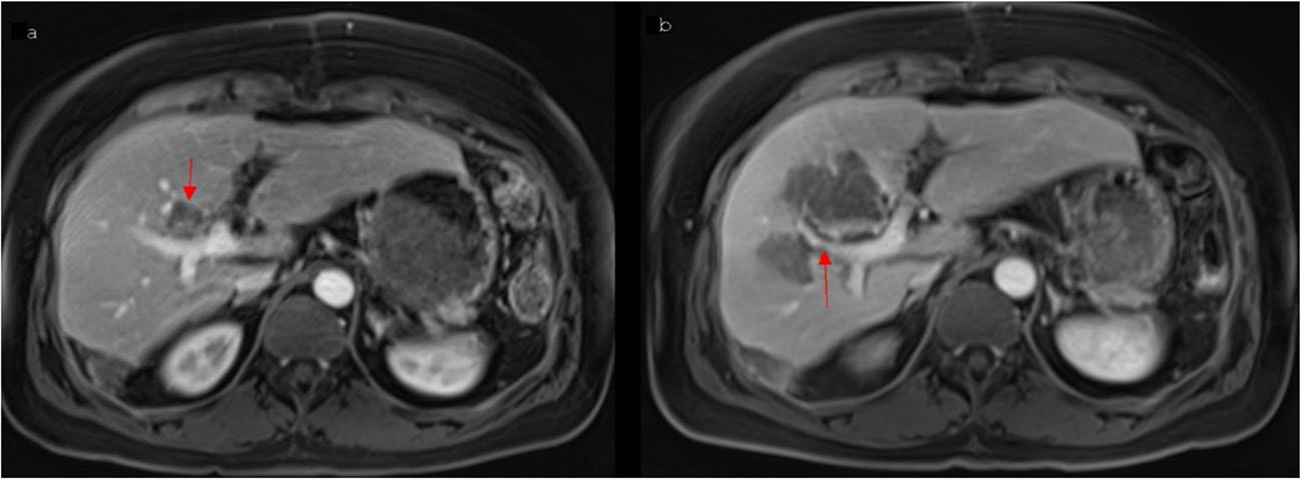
A 45-year old woman with a centrally located metastasis of colorectal cancer. (a) The Gd-EOB-DTPA-enhanced T1 vibe 3d fat suppressed magnetic resonance imaging in portal venous phase shows a centrally located metastasis (arrow) and a freely perfused right anterior branch of the portal vein. (b) 6 weeks after IRE imaging shows a newly occurred vessel narrowing of the portal vein branch (arrow).
